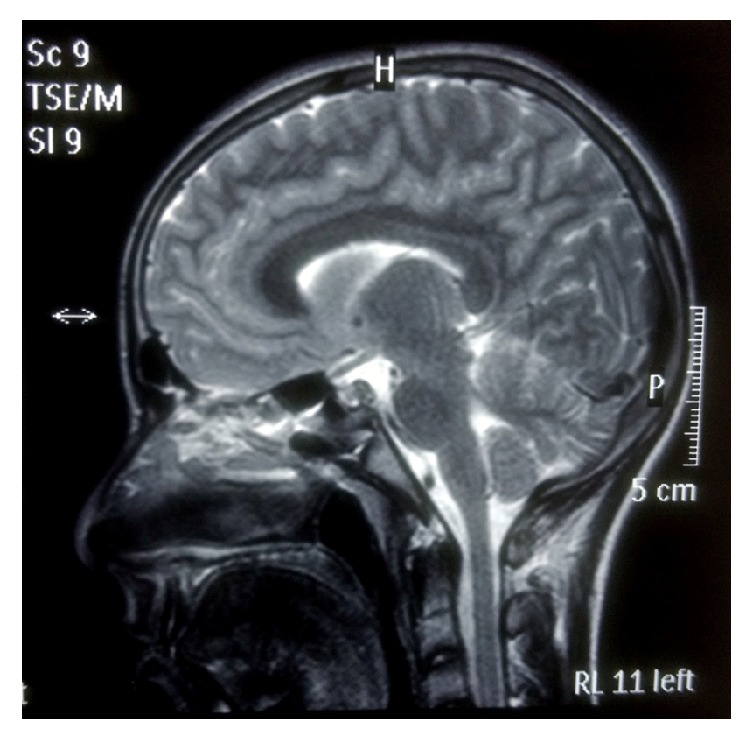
MRI head and orbit at the level of the midorbit and apex showing absence of any granulomatous lesions nor any extraocular muscle thickening (ruling out myositis or orbital pseudotumor).
